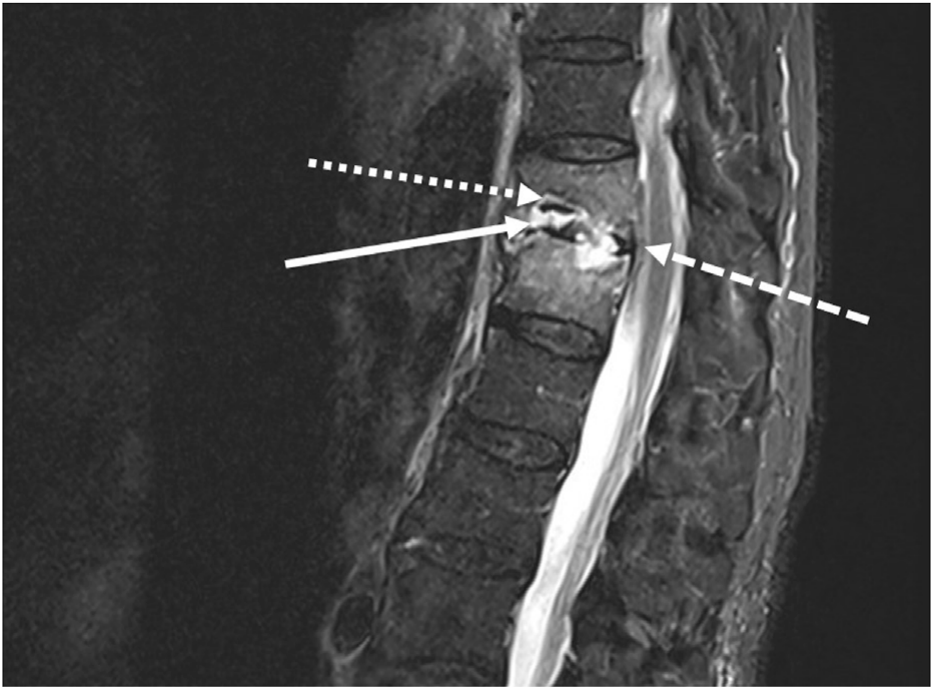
Medullar magnetic resonance imaging: Decreased height and STIR hypersignal of disc (white arrow) with irregularity and erosion of T11-T12 vertebral body endplates (white dotted arrow), and epidural collections (white dashed arrow).
